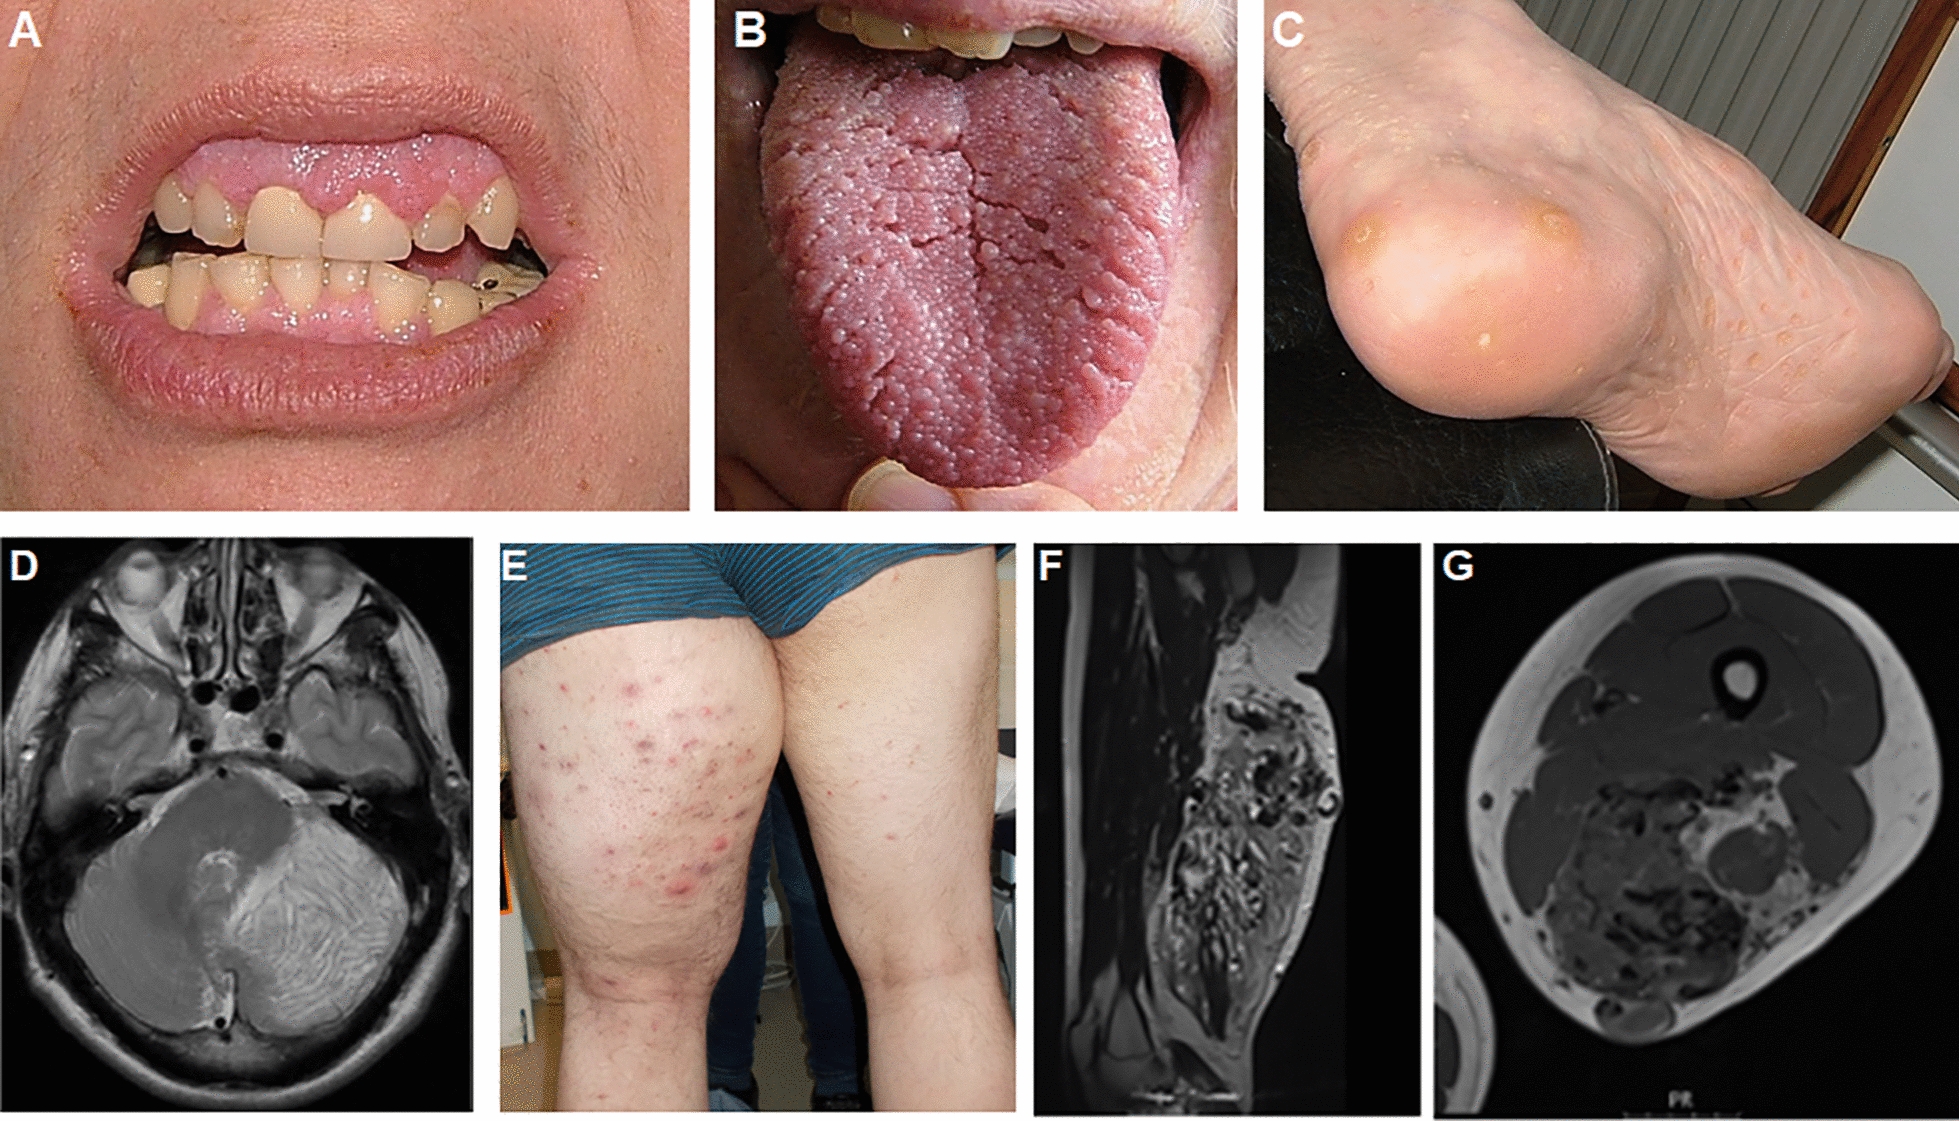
PHTS clinical manifestations. A Oral mucosal features. Gingival papillomatosis and neuromas may encroach on the dentition and cause difficulty with dental hygiene. B Papillomatosis of the tongue. C Plantar and palmar keratoses. Some are hyperkeratotic and may resemble viral verrucous lesions. D Axial T2 MRI image showing abnormal tissue in the left cerebellar hemisphere with the characteristic tigroid appearance and apparently preserved cerebellar folia of Lhermitte-Duclos Disease. E Segmental overgrowth of left thigh. This presented in adolescence, with no evidence of the lesion in early childhood. F, G MRI images demonstrating vascular malformation with fast and slow flow elements infiltrating muscle and fat
